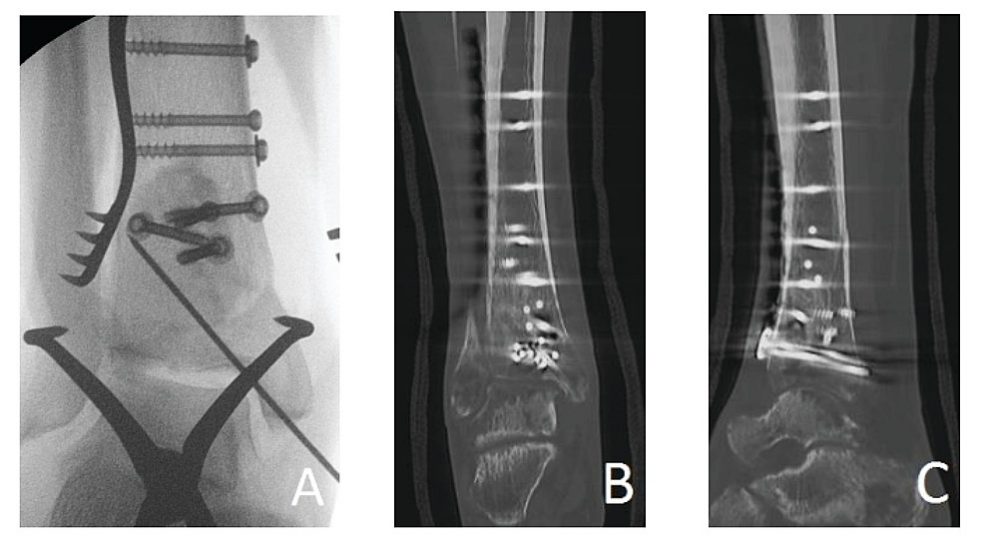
Signs of Cerament-G remodelling as seen in CT scan. A: Cerament-G as a void filler intraoperatively; B: CT scan coronal view at six months postoperatively; C: CT scan sagittal view at six months postoperatively.
